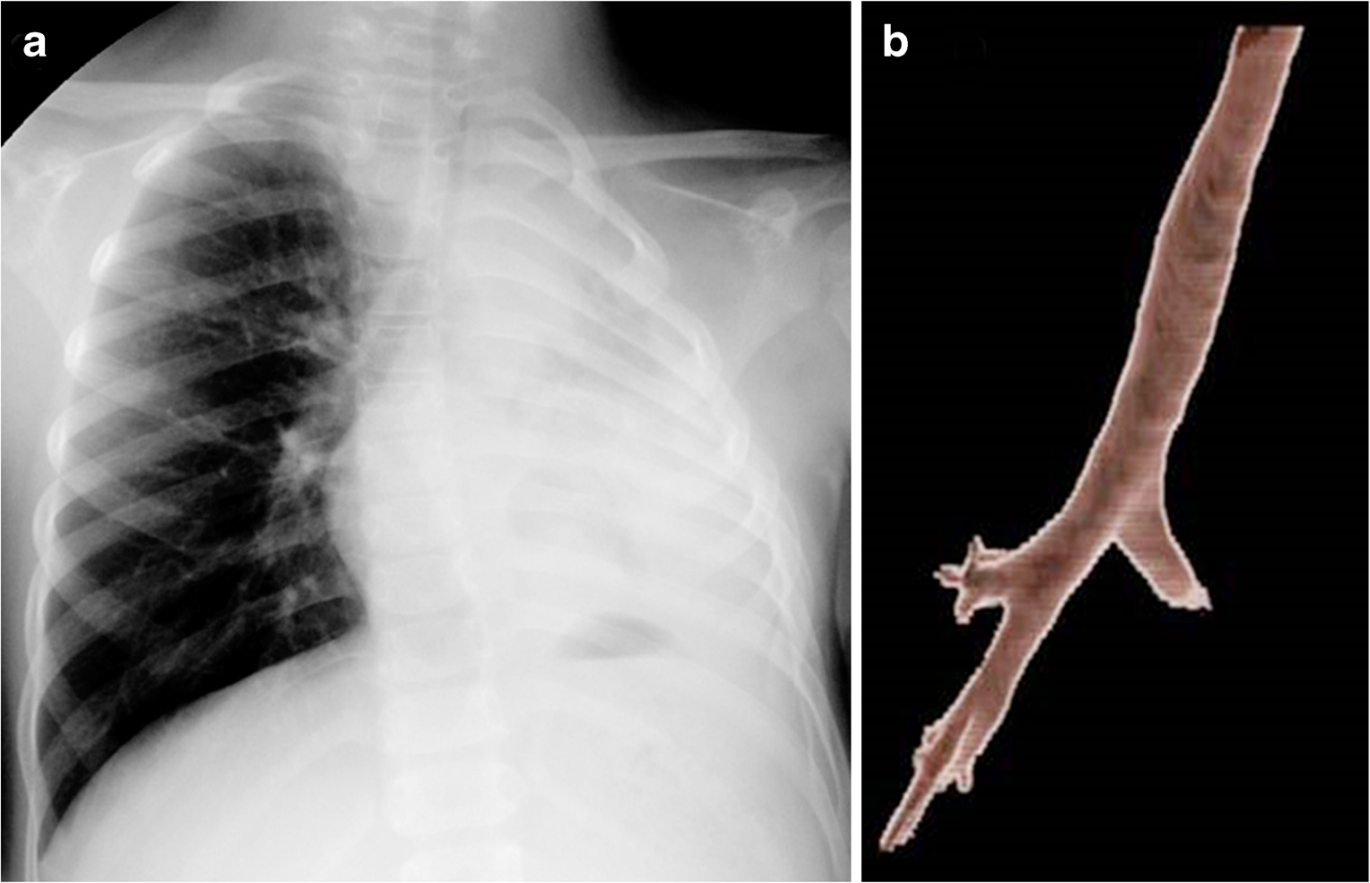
Primary progressive lymphobronchial tuberculous disease in a 6-year-old boy. a Anteroposterior chest radiograph shows left lung collapse. b Volume-rendered CT shows occlusion of the left mainstem bronchus caused by lymphobronchial disease
